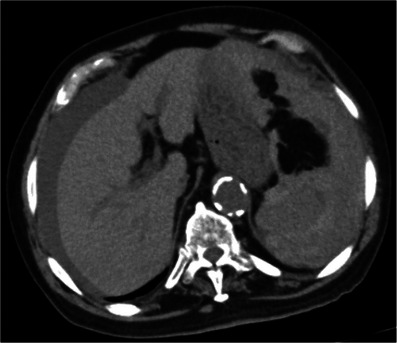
The plain abdominal computed tomography after the colonoscopy. The plain abdominal computed tomography revealed high attenuation ascites but their cause was unclear.
